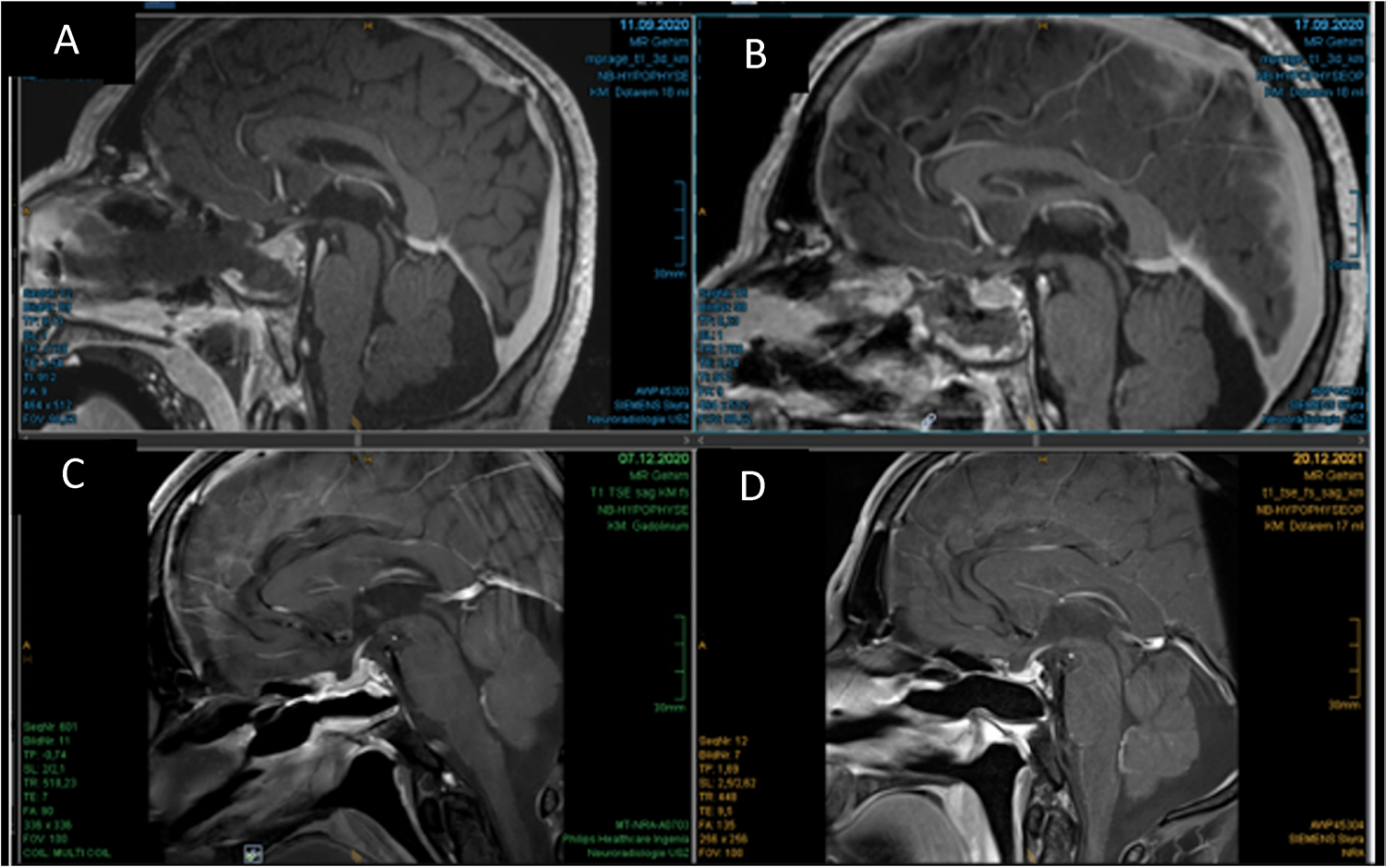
MRI follow-up patient 1 showing smoothening of the sellar wall over time. No signs of infection, swelling, or other pathological reactions were observed. A Intraoperative MRI (no Liqoseal), B day 6 postoperatively, C 3 months postoperatively, and D 15 months postoperatively
